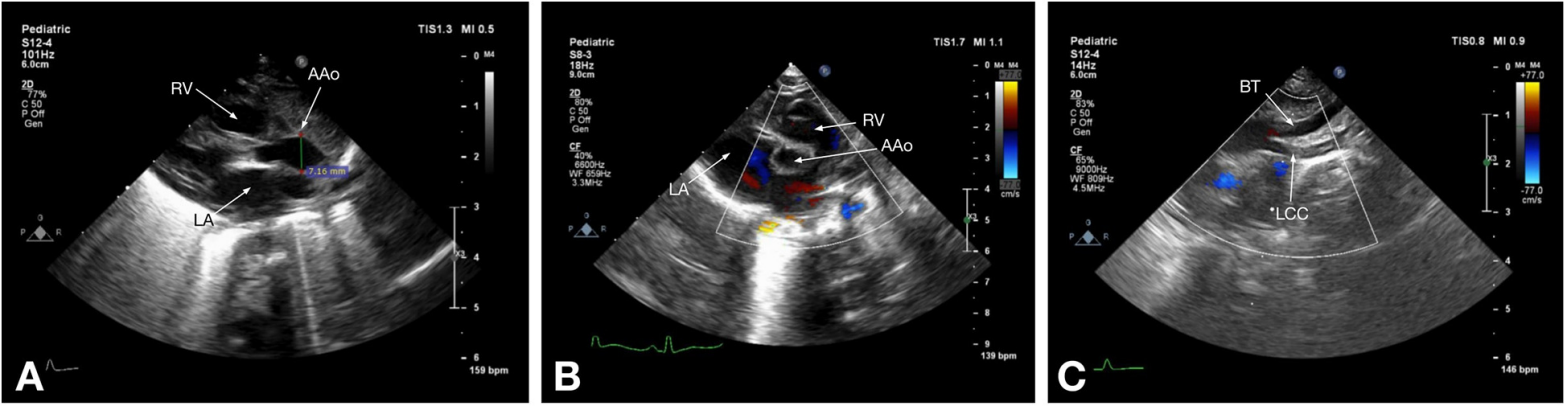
Echocardiographic images of a 7-day-old patient with type B IAA: (A) parasternal long-axis view shows the right ventricle (RV) and left atrium (LA) and the diameter of the ascending aorta (AAo) above the sinotubular junction; (B) parasternal short-axis view shows the right ventricle (RV), left atrium (LA), and the ascending aorta (AAo); (C) suprasternal notch view shows the brachiocephalic trunk (BT) and the left common carotid artery (LCC), followed by the echo signal disruption, corresponding to type B IAA.
